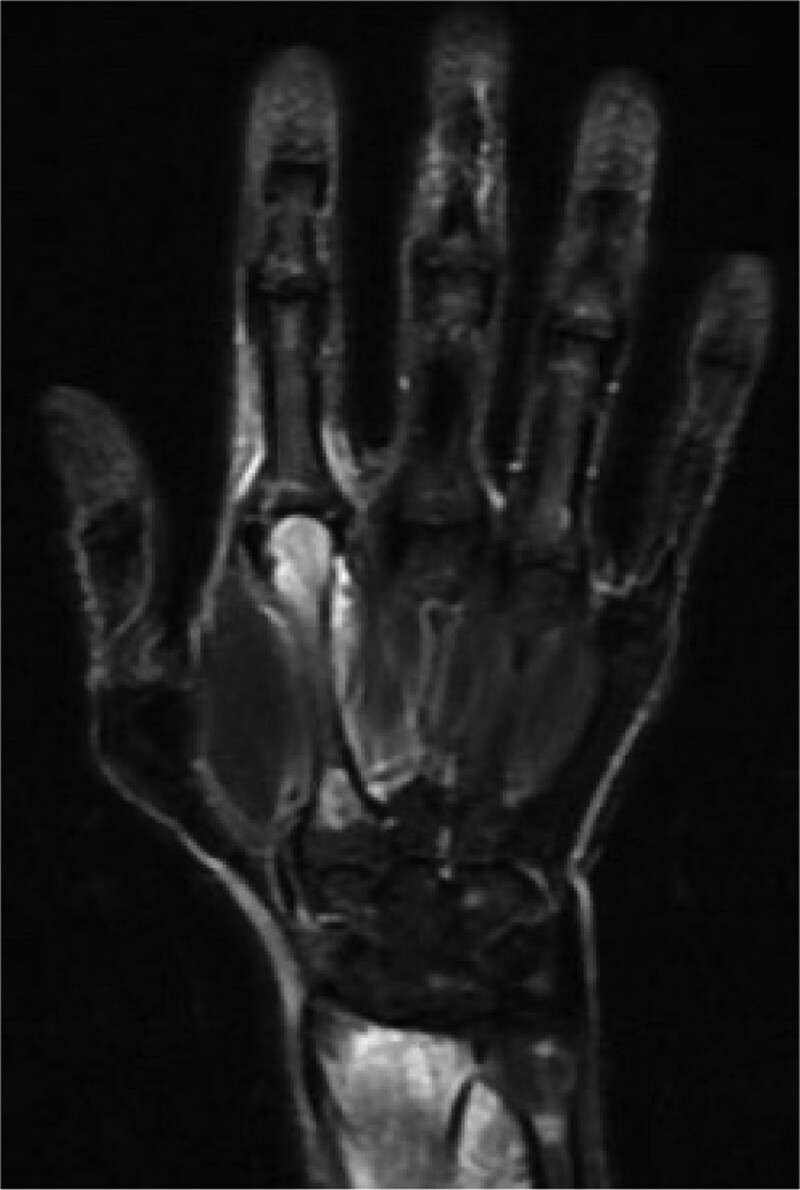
Plain magnetic resonance imaging (short T1-inversion recovery mode) of the right hand conducted 1 month before starting antibiotic treatment. The radius, ulna, and second metacarpal bone show high intensity.
